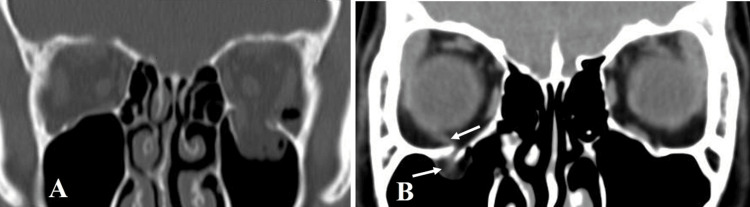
Typical CT images of an orbital floor blow-out fracture. (A) The left orbital floor bone is punched out outwardly and orbital soft tissues fall into the maxillary sinus. Emphysema presents within the left orbit. (B) Linear fracture of the right orbital floor bone with strangulation of the orbital contents, including the inferior rectus muscle (→). Emergency surgery is indicated for such cases.
